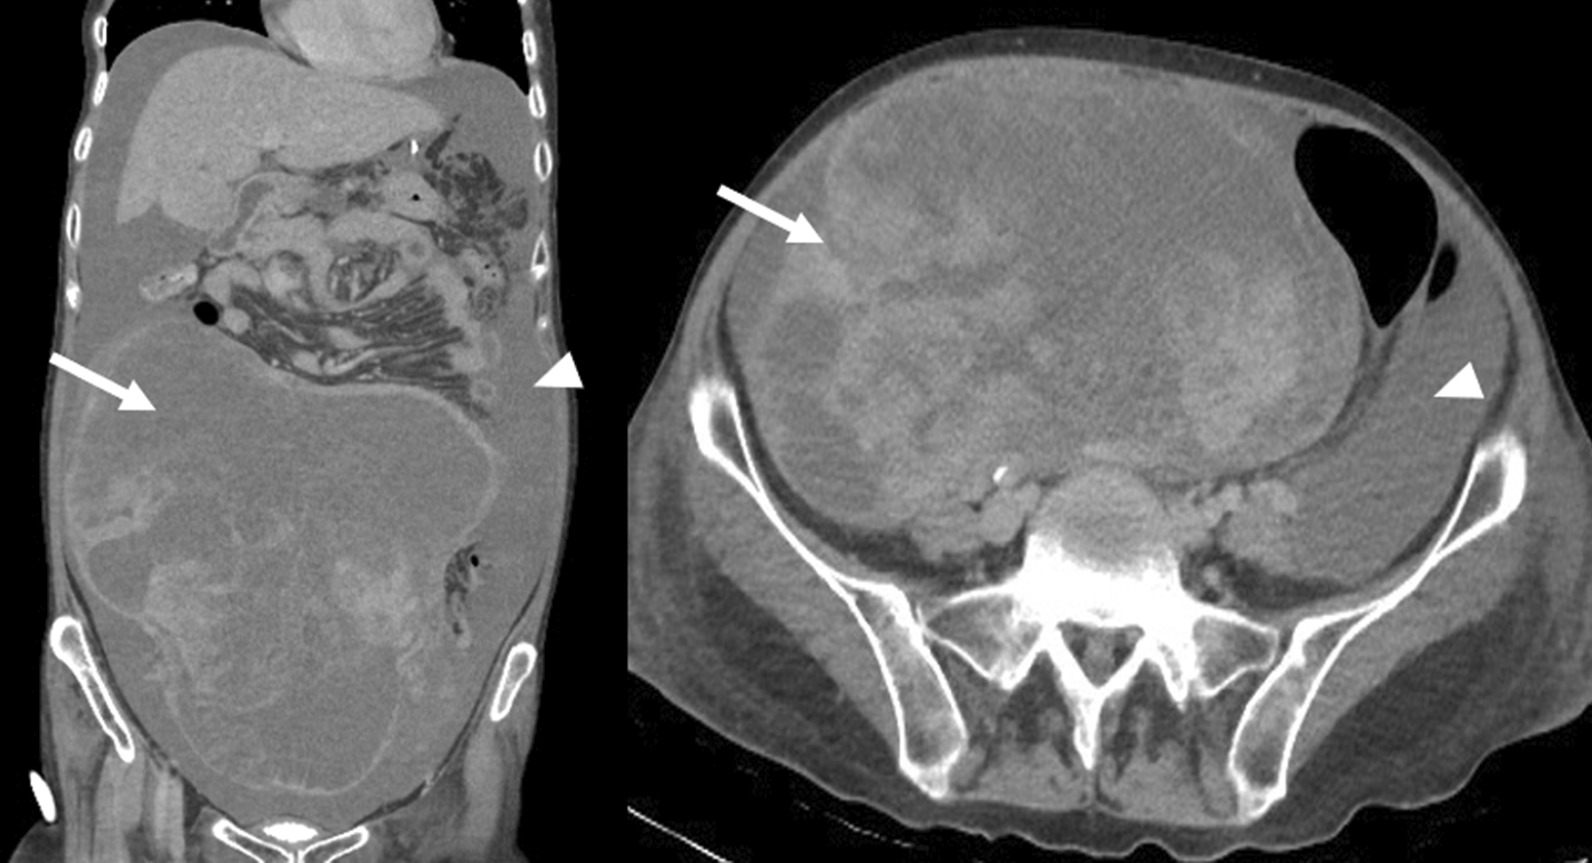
Computed tomography scan showed a 30-cm-sized irregularly hyperenhancing mass (arrow) with large amount of ascites (arrow head) and peritoneal thickening suggesting ovarian malignancy with peritoneal carcinomatosis
